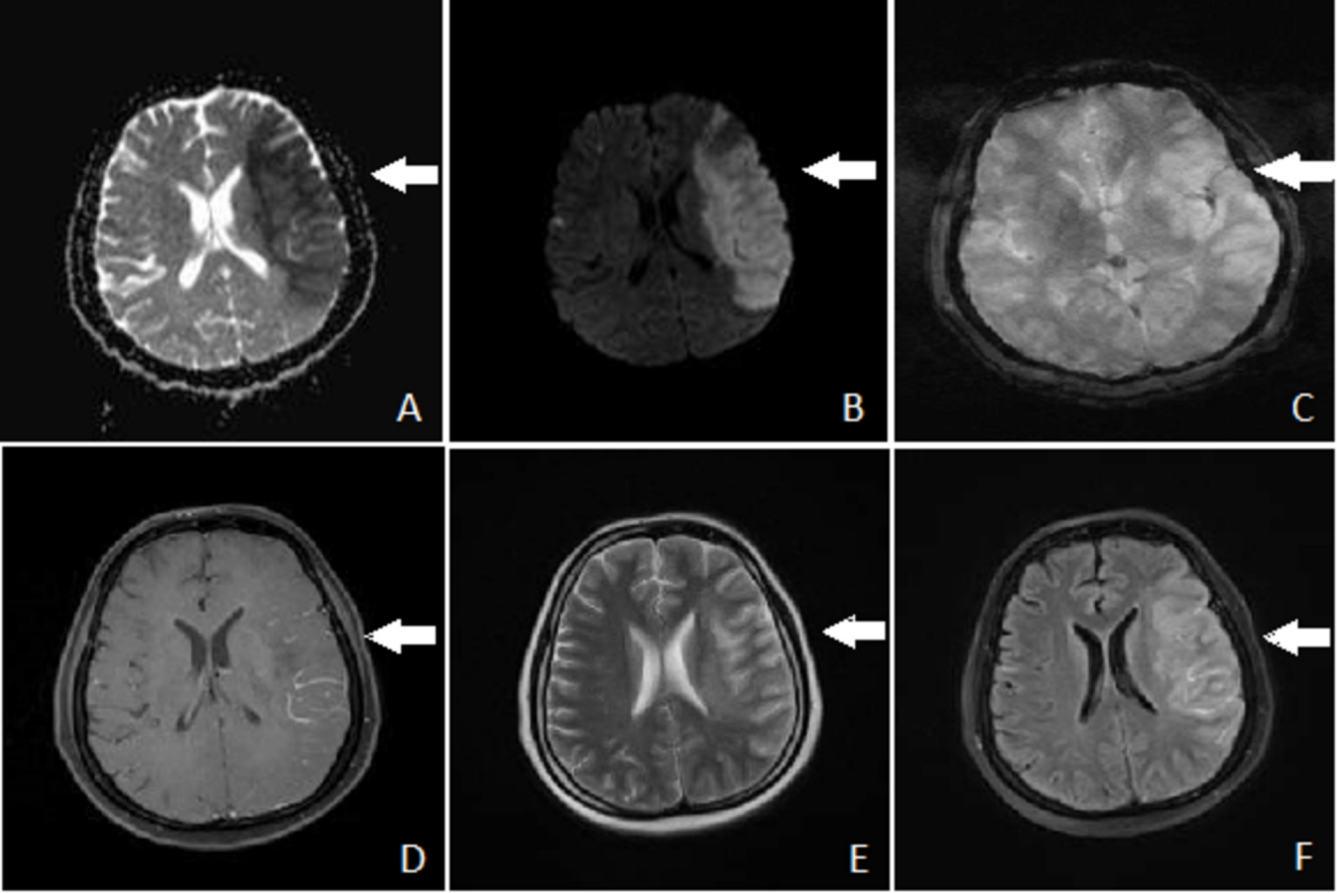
MRI scan of head in axial view (A) Apparent diffusion coefficient (ADC) scan showing low signals in a large area of left parietal region. (B) Diffusion weighted 1 (DW1) scan showing a large area of restricted diffusion in left parietal region. (C) Susceptibility weighted 1 (SW1) scan showing a small area of blooming in left parietal region suggesting hemorrhage. (D) Contrast-enhanced T1 scan showing subtle gyriform enhancement in left parietal region. (E) T2 scan showing abnormal high signals in left parietal region. (F) Fluid-attenuated inversion recovery (FLAIR) scan showing abnormal high signals in left parietal region with perifocal edema obscuring grey white interface.
